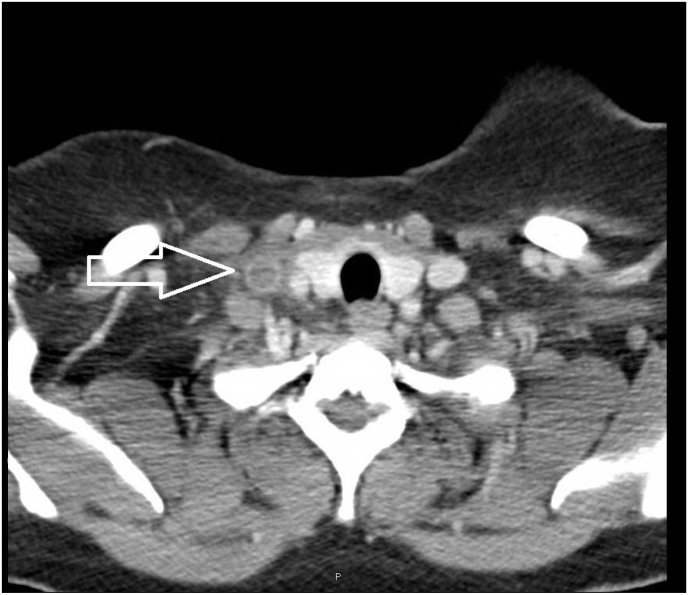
Contrast-enhanced computed tomography showing thrombosis in the right internal jugular vein.
